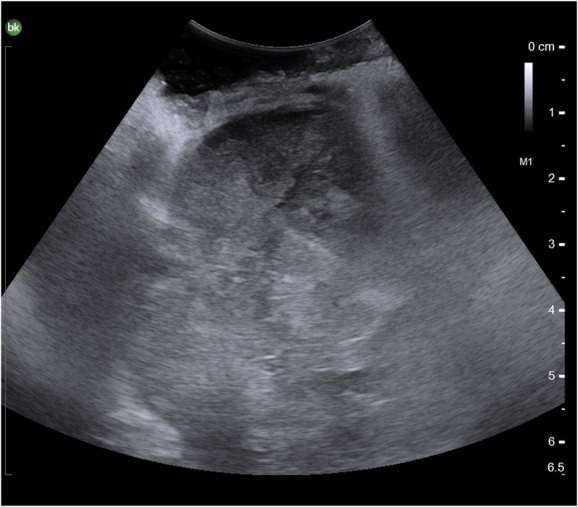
Intraoperative ultrasound for visualization of the tumor margins.
